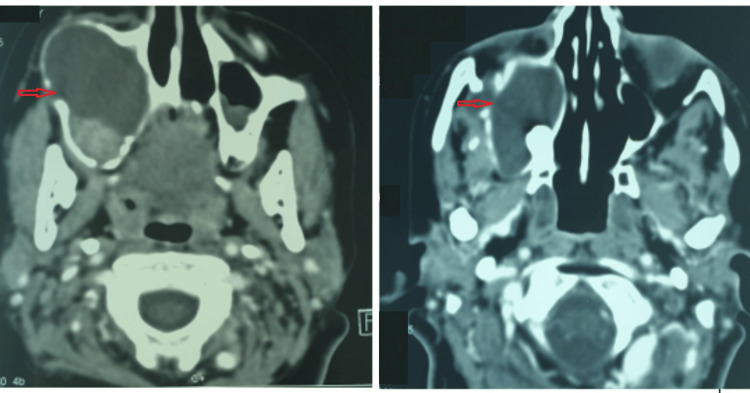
Axial CT showing a massive cystic lesion invading the entire right maxillary sinus with osseous erosion Red arrow: Cystic lesion invading the entire right maxillary sinus
